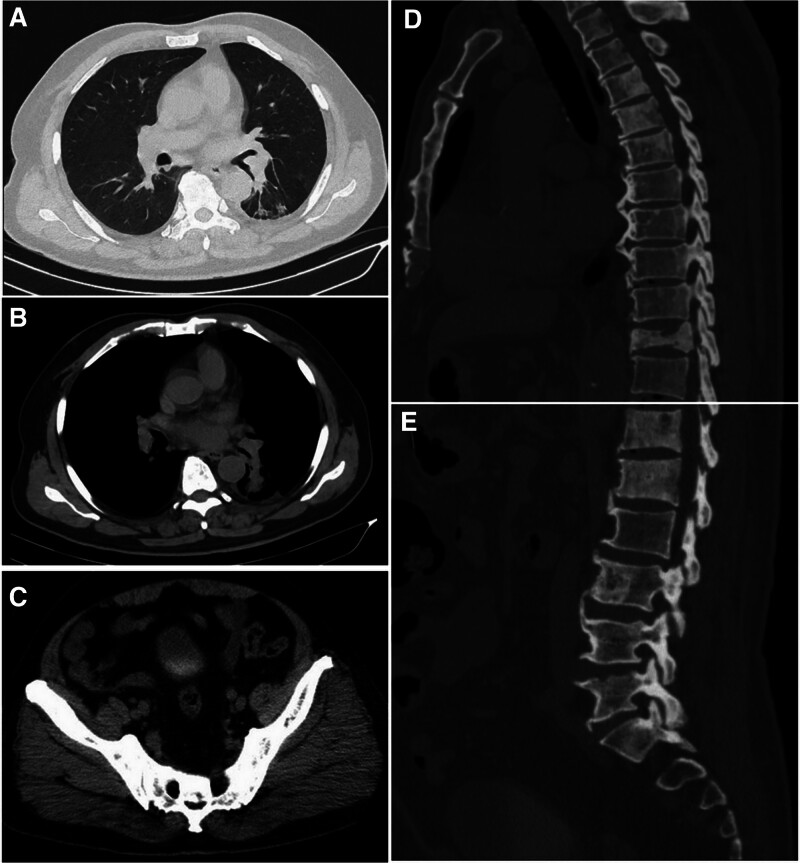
A 16-slice computed tomographic scan revealed that the left lung space-occupying lesion with obstructive pneumonia was almost completely absorbed, and the osteolytic destruction had disappeared. (A) Lung window; (B) mediation window; (C) right ilium; (D) thoracic vertebra; (E) lumbar vertebra.
